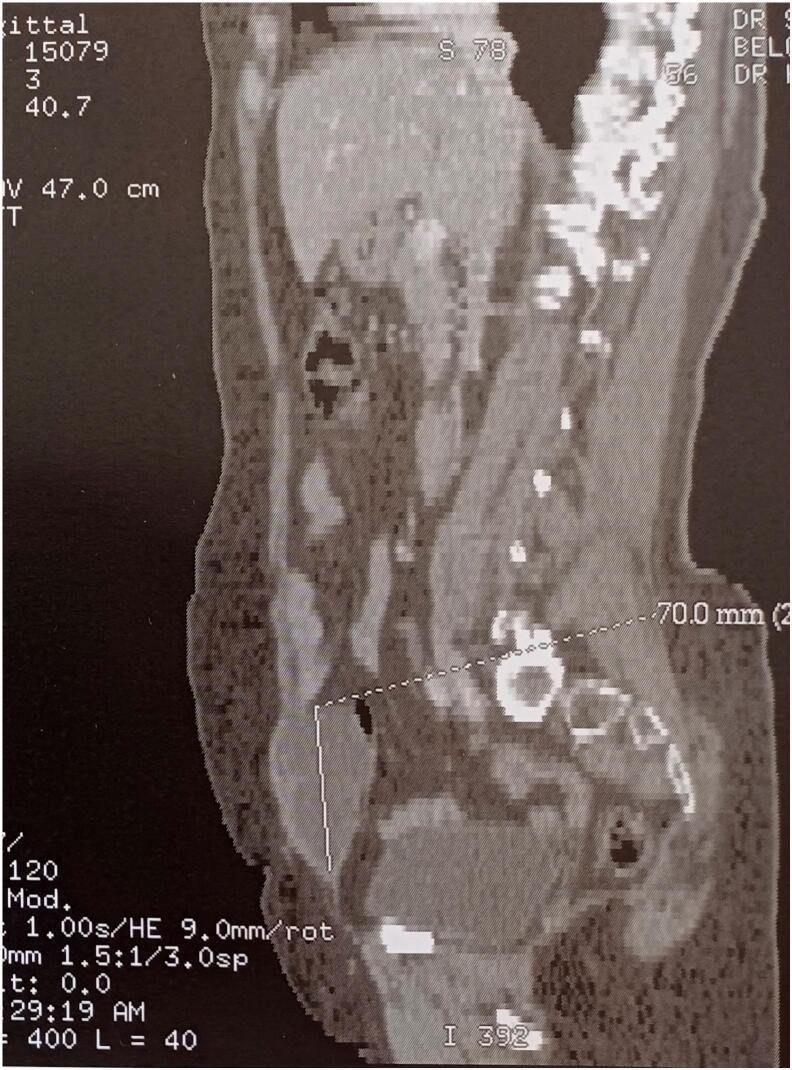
Axial CT scan displayed a solid-cystic parietal formation within the rectus abdominis muscle, positioned below the umbilicus, with a budding tissue portion measuring 50 × 40 × 70 mm.
